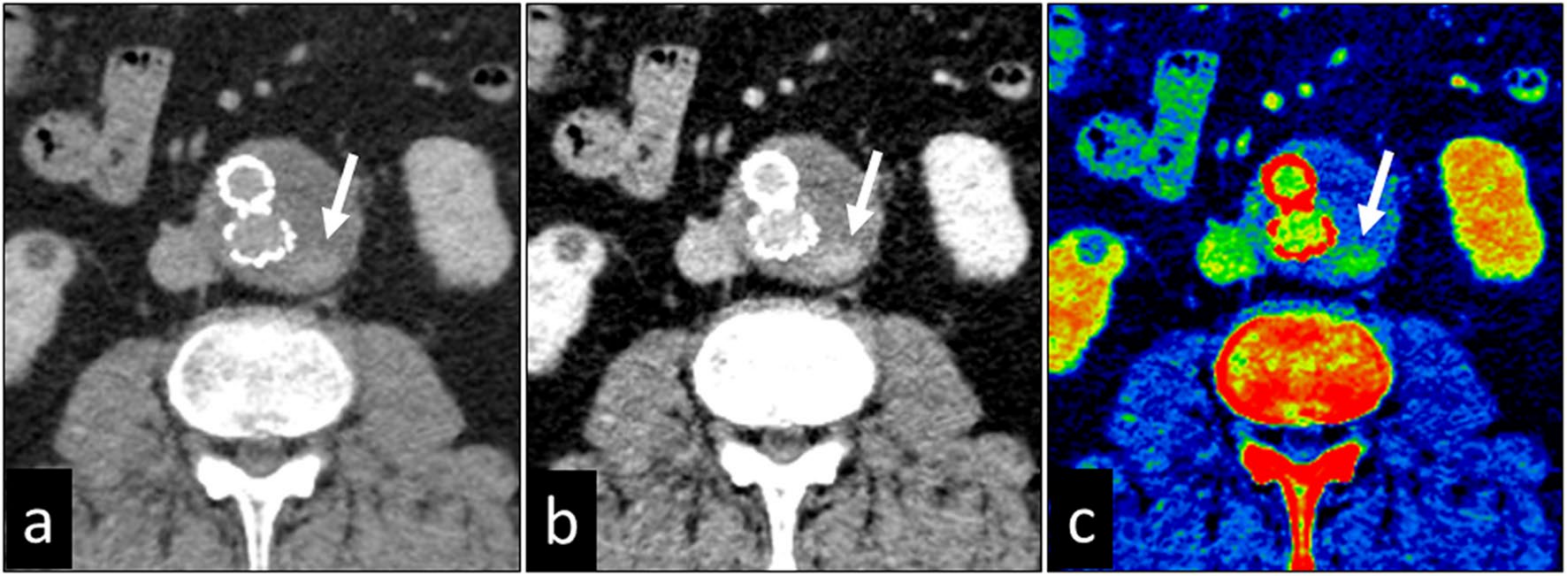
A 75-year-old man with endoleak after endovascular aortic repair. Virtual monochromatic images at 70 keV (a), 40 keV (b), and a color image of the iodine map (c) obtained with CT angiography are shown. The vessel contrast and the endoleak delineation (arrow) are better on the 40 keV image and the iodine map than on the monochromatic 70 keV image
